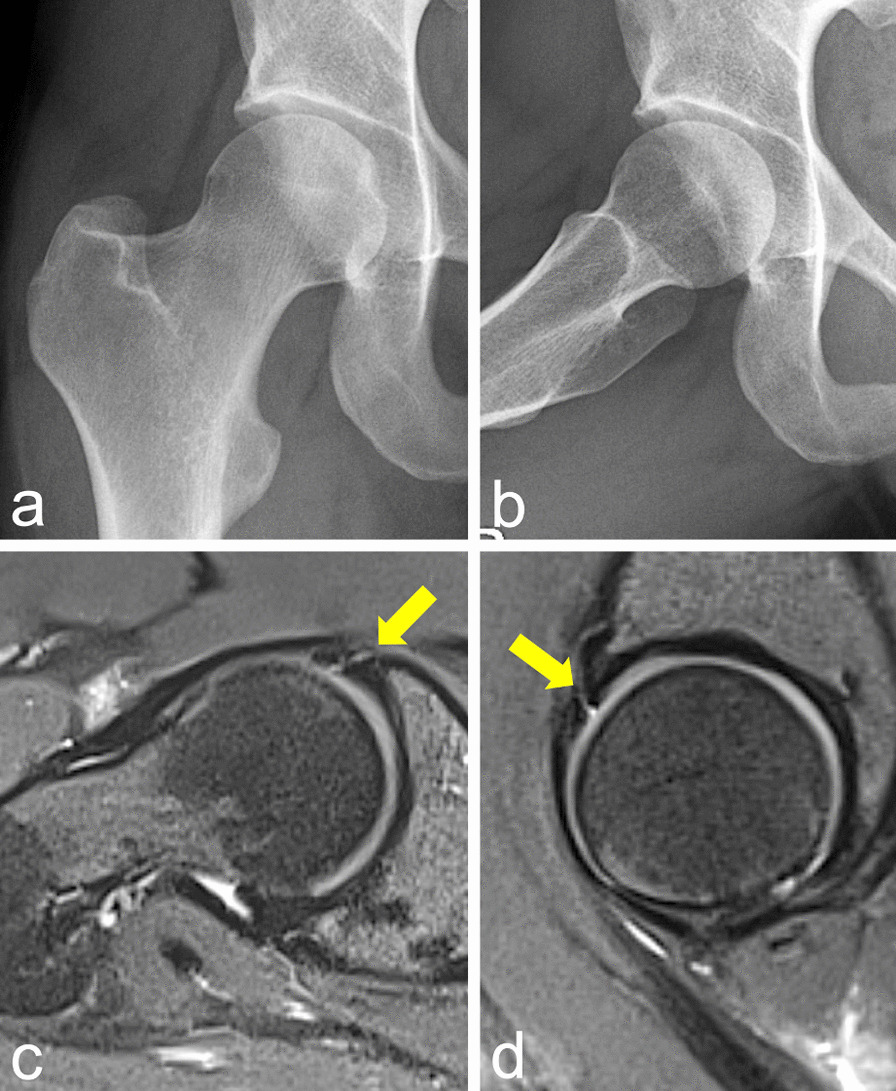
a–d Preoperative imaging of right hip. a, b Pelvis anteroposterior radiograph and frog-lateral view with mild cam deformity and borderline pincer deformity. c, d High-resolution axial and sagittal magnetic resonance imaging with labral tear at the anterolateral acetabulum (arrow)
